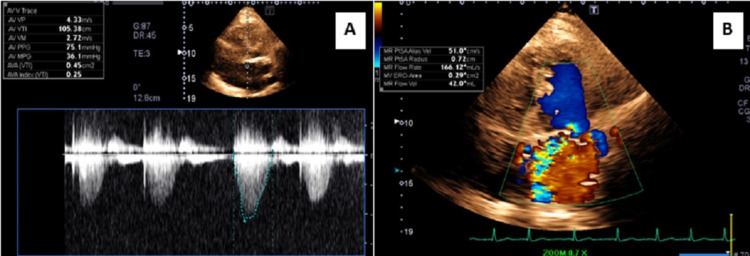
Echocardiogram showing severe aortic stenosis (A). Continuous-wave Doppler in apical five-chamber view showed a peak aortic jet velocity of 4.3 m/sec with the calculated aortic valve area of 0.45 cm2. (B) Color Doppler in apical four-chamber view revealed severe mitral regurgitation. The eccentric regurgitant jet was noted to be selectively directed towards the right pulmonary veins.
